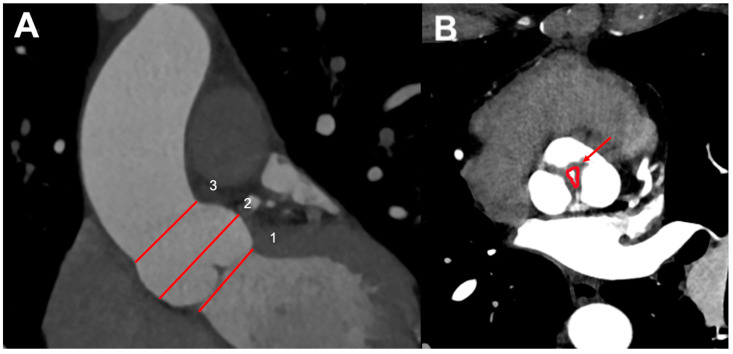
Measurements of aortic root at different levels by CT (A): (1) aortic valve annulus (the hinge points of the valve cusps); (2) midpoint of the sinuses of Valsalva; and (3) sinotubular junction. (B) CT planimetric measurement of aortic regurgitant orifice (ARO) (arrow).
